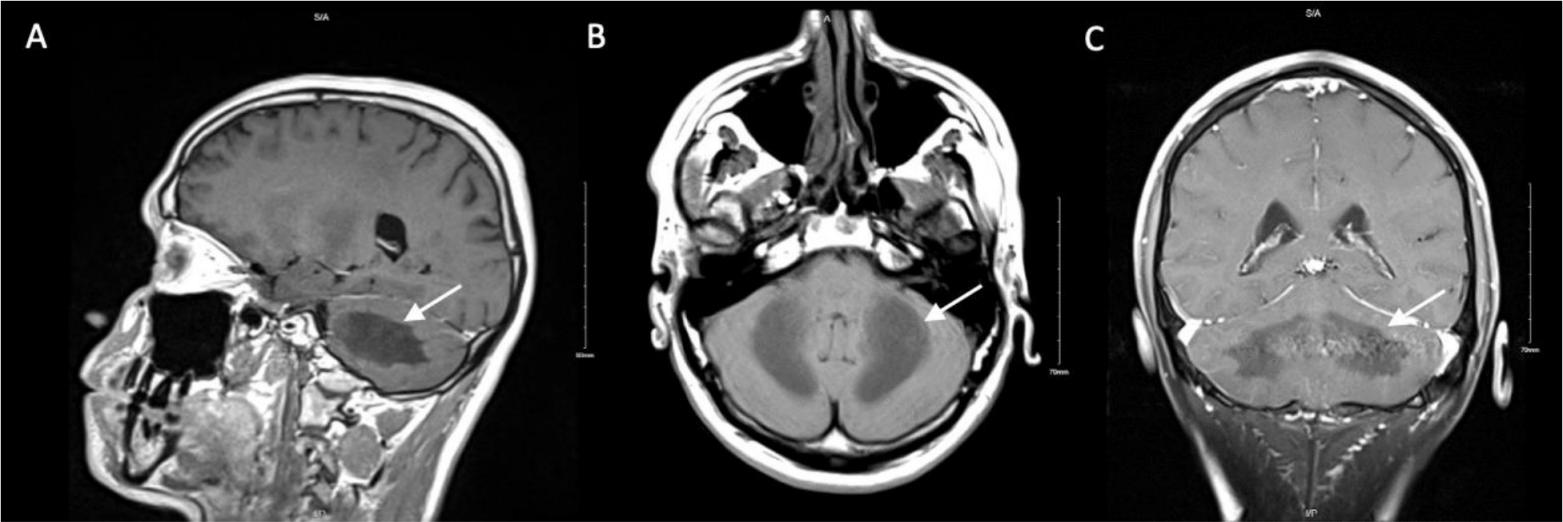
Brain magnetic resonance imaging (MRI). (A) Sagittal (B) Axial (C) Coronal images demonstrate symmetric abnormal degeneration in the cerebellar white matter extending to the posterior limbs of the internal capsule. Differential diagnosis includes acquired toxic/metabolic degenerative disorder.
